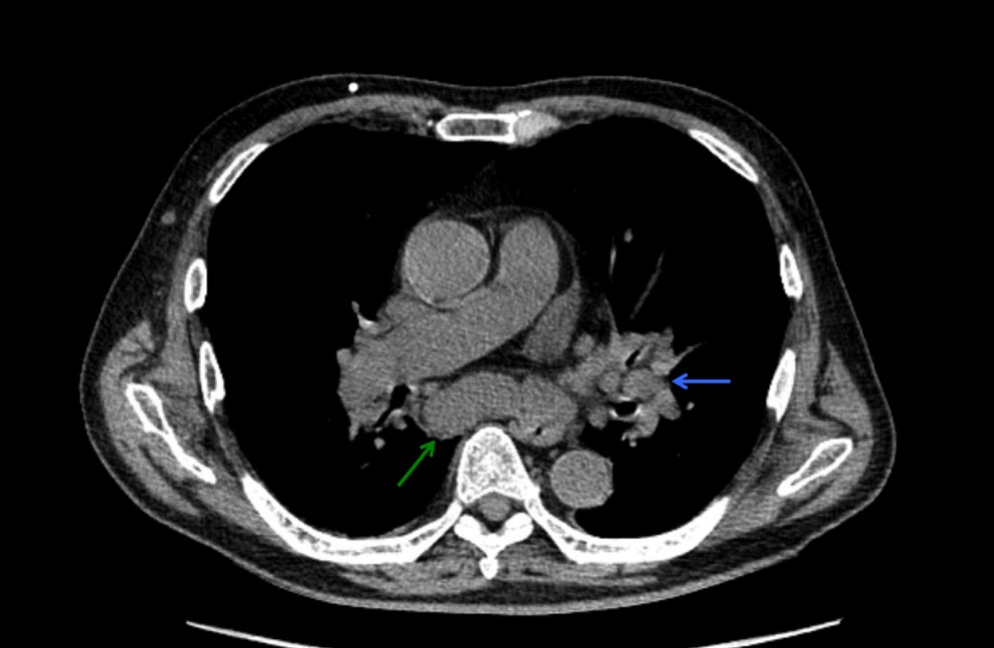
Chest computed tomography on day 21. Marked enlargement of the subcarinal (green arrow) and hilar (blue arrow) lymph nodes observed
